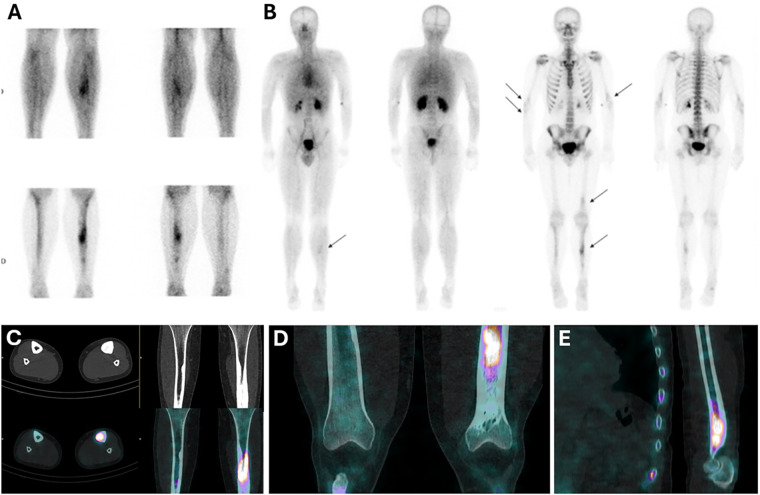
Bone scintigraphy showing hyperemia (A) and hyperfixation in the tibias, left femur, and humeri (B), associated with intramedullary osteosclerosis on CT scans (C–E).
